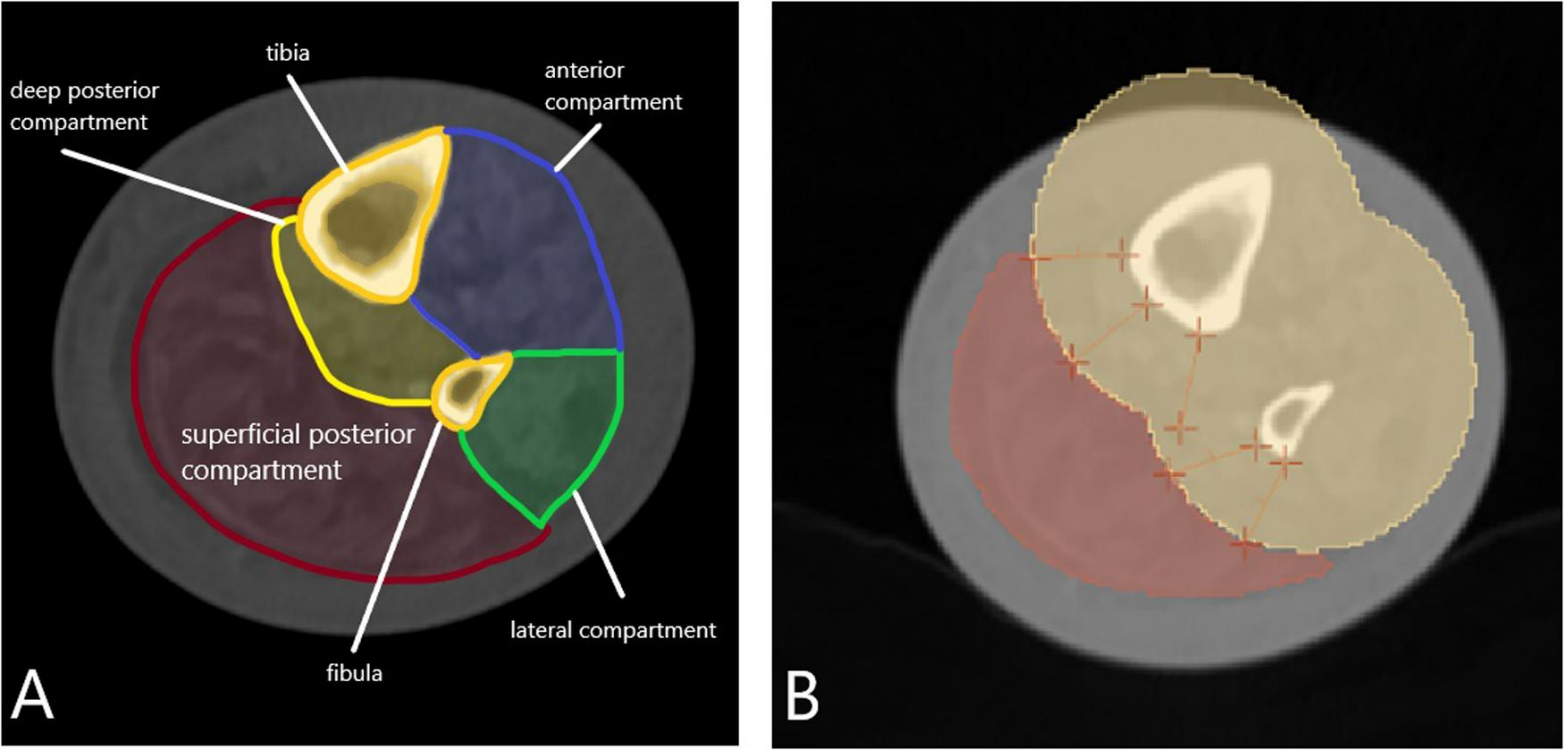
Left (affected) leg from the first (preintervention) scan of the first patient. The compartments of the lower leg are visualized at 60% of its length, measured from the distal end of the medial malleolus to the knee joint space, using low-dose CT. (A) The superficial posterior compartment—containing the gastrocnemius and soleus muscles—is defined as the region of interest (ROI). (B) Tibial and fibular bones are shown in yellow, surrounded by a 20-mm safety margin to correct for potential patient motion. The red area indicates the calf muscles of the superficial posterior compartment. All muscle tissue posterior (dorsal) to the bones, including the margin, was delineated as the volume of interest (VOI) for the muscle contour VOI. Yellow = bones + margin; red = muscle compartment.
